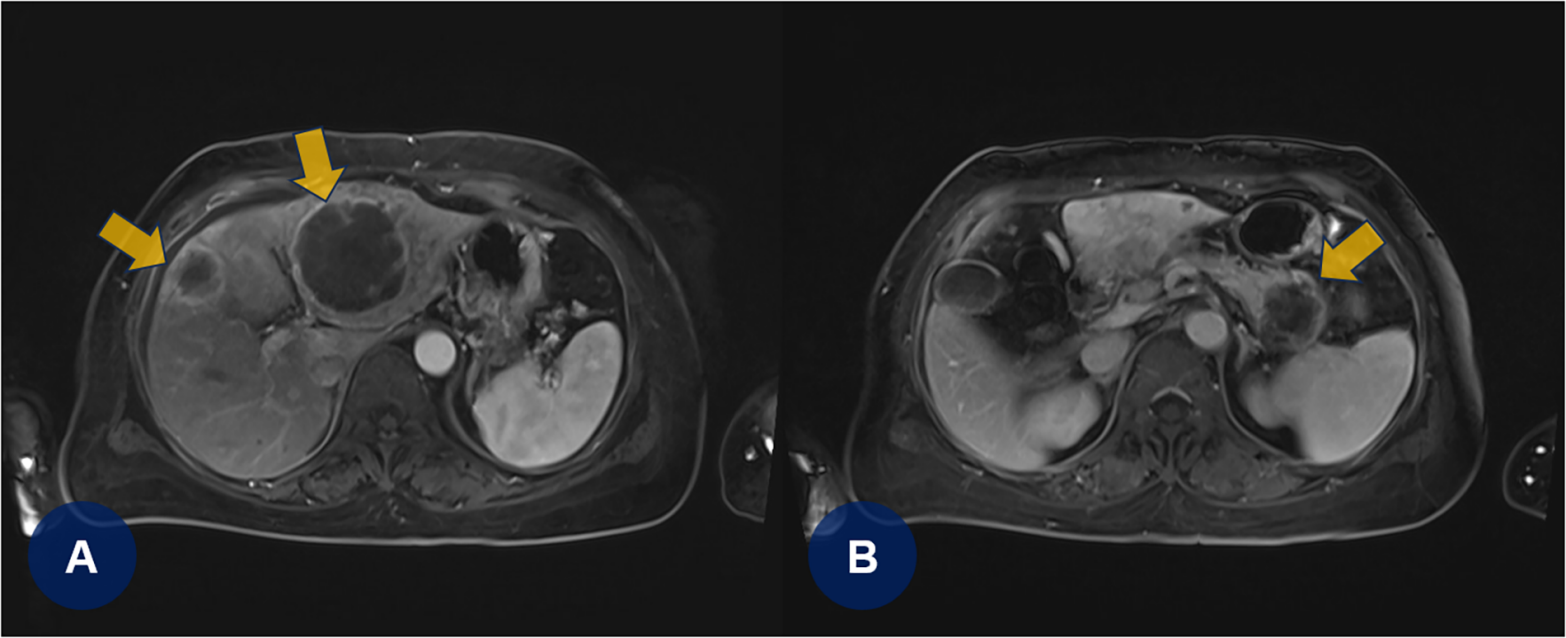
(A,B) Dynamic contrast-enhanced magnetic resonance imaging confirms primary pancreatic cancer with hepatic metastases (yellowish arrows).
